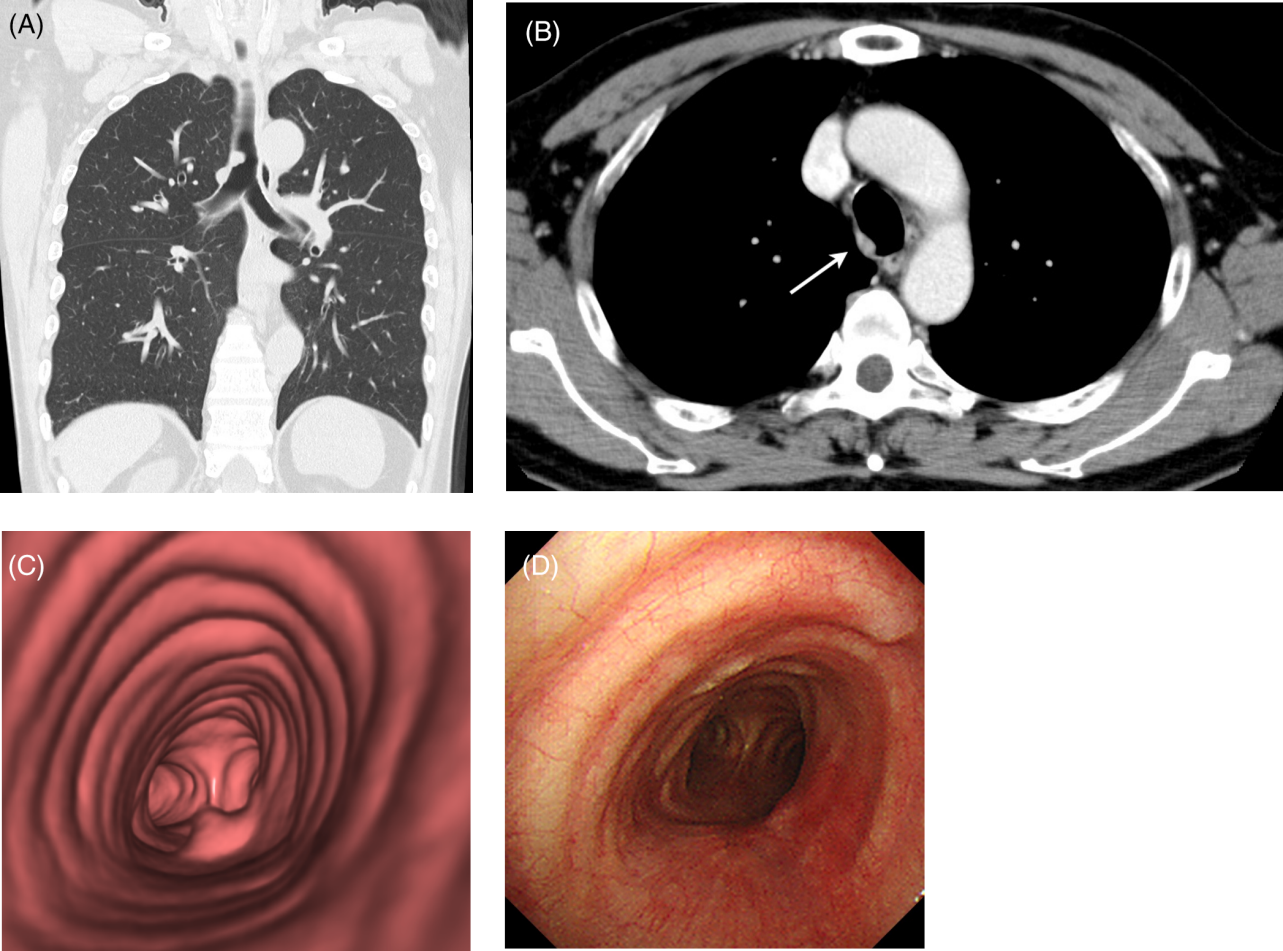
(A and B) Coronal computed tomography (CT) and virtual bronchoscope images of the chest demonstrates a 1.5 cm mass protruding into the lower trachea before endoscopic treatment. (C) The lesion shows strong contrast enhancement similar to that of blood vessels on post‐contrast axial CT before endoscopic treatment. (D) Image from bronchoscopy performed 3 months after endoscopic removal of the lesion reveals no obvious abnormalities.
